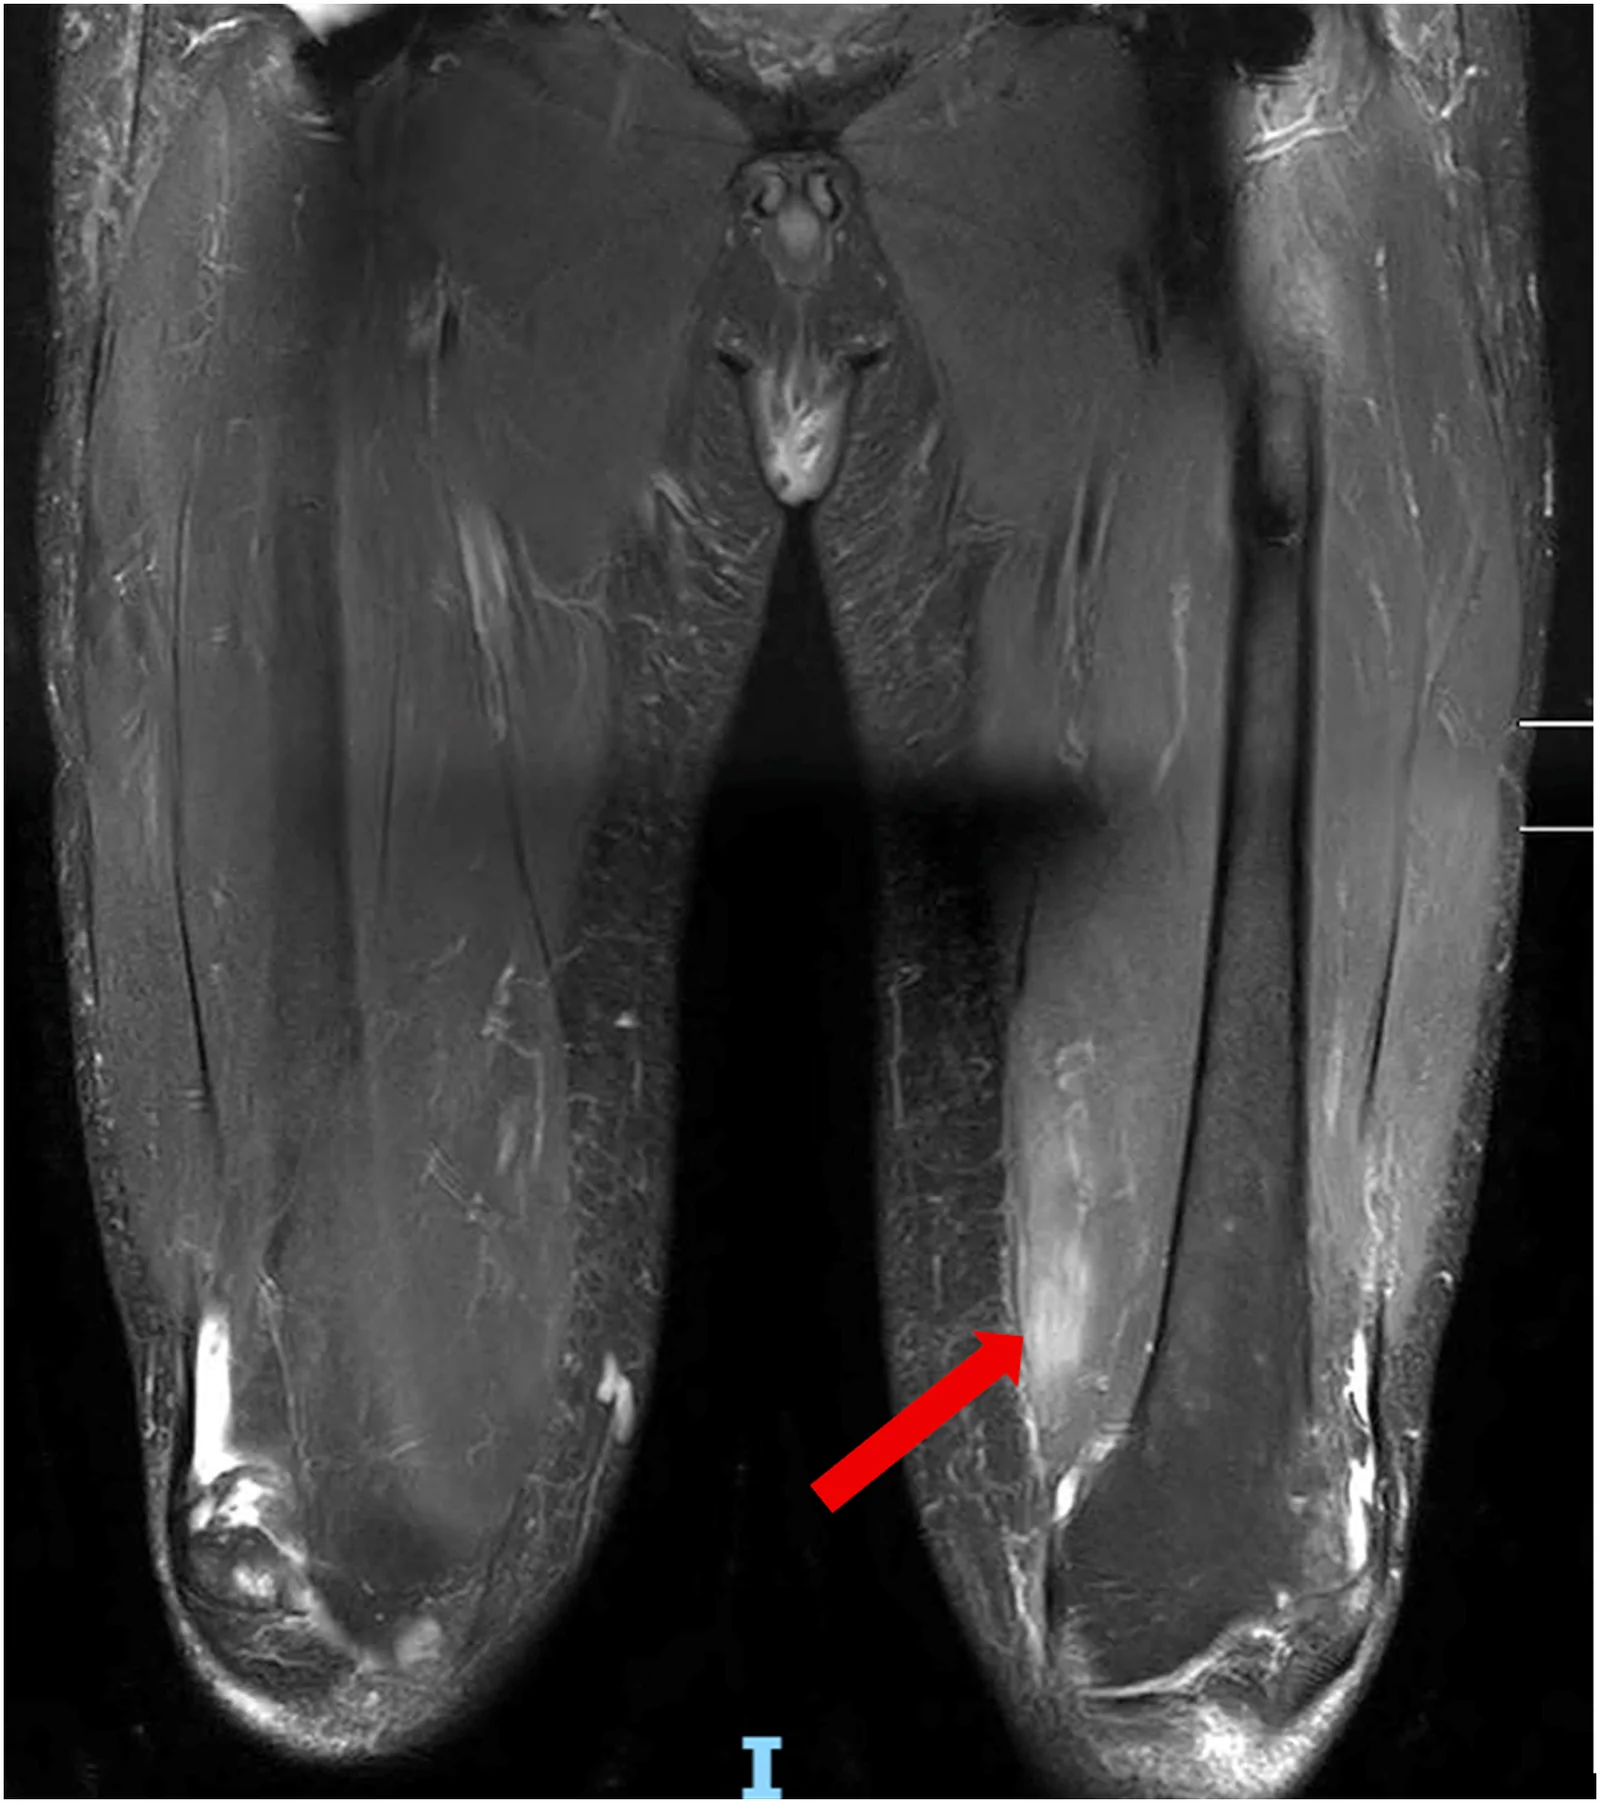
MRI of Skeletal Muscle Magnetic resonance imaging (MRI) of skeletal muscle demonstrating symmetric short tau inversion recovery hypersensitivity involving proximal lower limb musculature, consistent with an inflammatory myopathic pattern (arrow).
